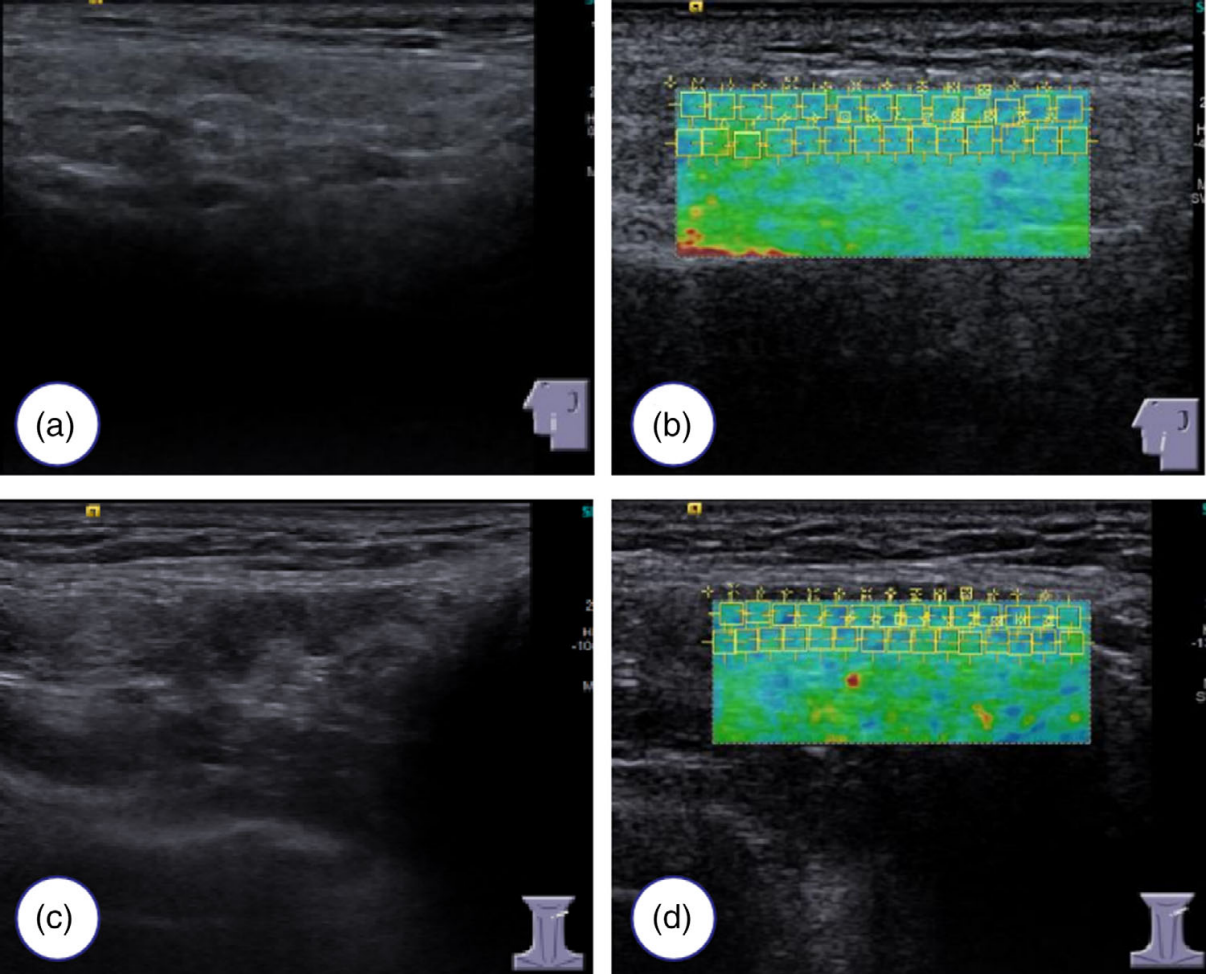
Ultrasonographic features and shear wave elastography of parotid and submandibular glands in patients with in the secondary Sjögren's Syndrome (sSS) Group. (a) Two‐dimensional ultrasonography of the parotid glands. (b) The shear wave velocity (SWV) of the parotid gland is 2.26 m/s. (c) Submandibular gland two‐dimensional ultrasonography. (d) The SWV of the submandibular gland is 2.32 m/s.
